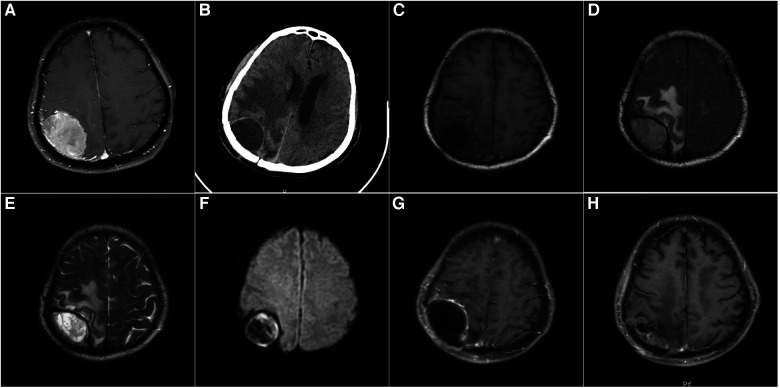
(A): A 68-year-old male patient with contrast-enhanced MRI: right apical meningioma, who underwent craniotomy for tumor resection. Postoperative pathology revealed atypical meningioma, and postoperative radiotherapy was performed. (B): Four months after the operation, the patient's left limb was numb and weak, without fever, and the skin flap showed no inflammation. CT showed a cystic lesion in the right parietal lobe. (C) T1-weighted MRI showed a hypointense lesion in the right parietal lobe. (D): FLAIR sequence of MRI showed a hyperintense lesion in the right parietal lobe. (E): T2-weighted MRI showed a hyperintense lesion in the right parietal lobe. (F) Diffusion-weighted MRI (DWI) showed a hyperintense lesion in the right parietal lobe. (G): Enhanced MRI showed a cystic lesion in the right parietal lobe with enhancement of the cyst wall. (H): The epidural abscess was removed, and the bone flap was preserved. After 5 months of surgery, MRI reexamination showed no recurrence of epidural abscess.
